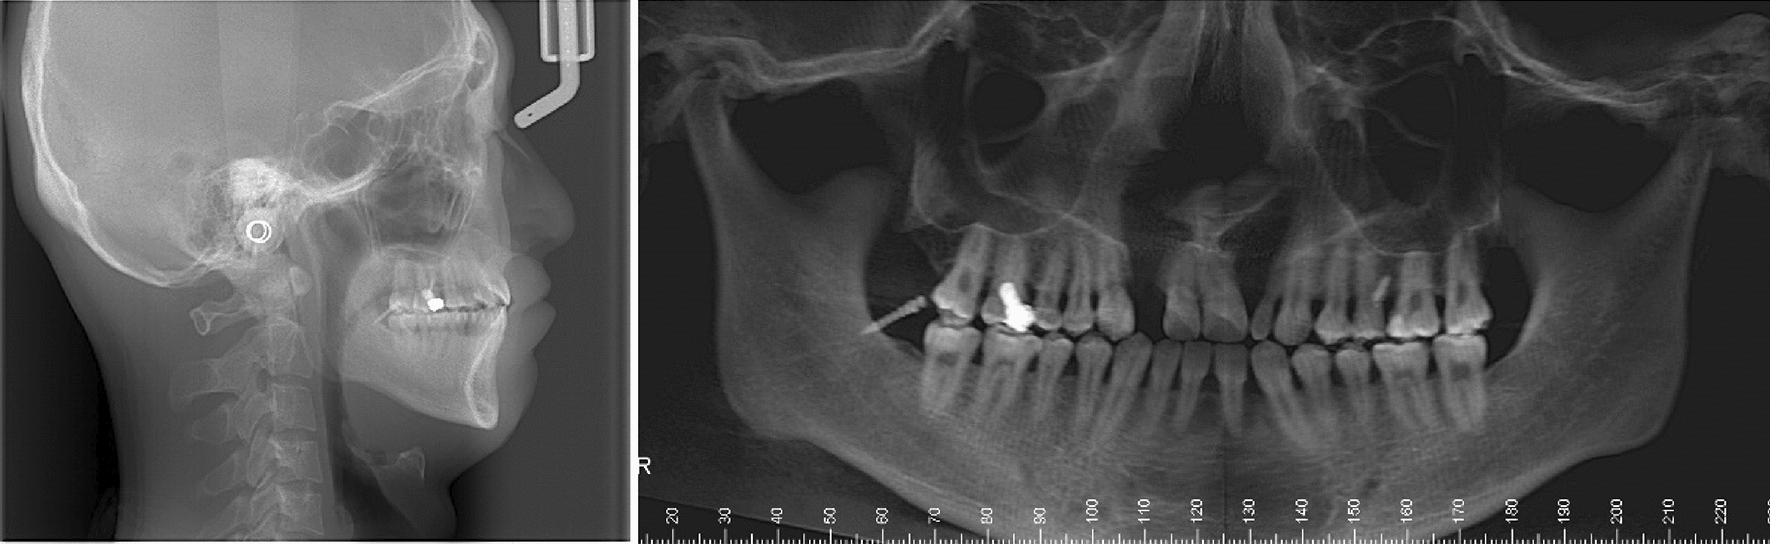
Posttreatment lateral cephalogram and panoramic radiograph
